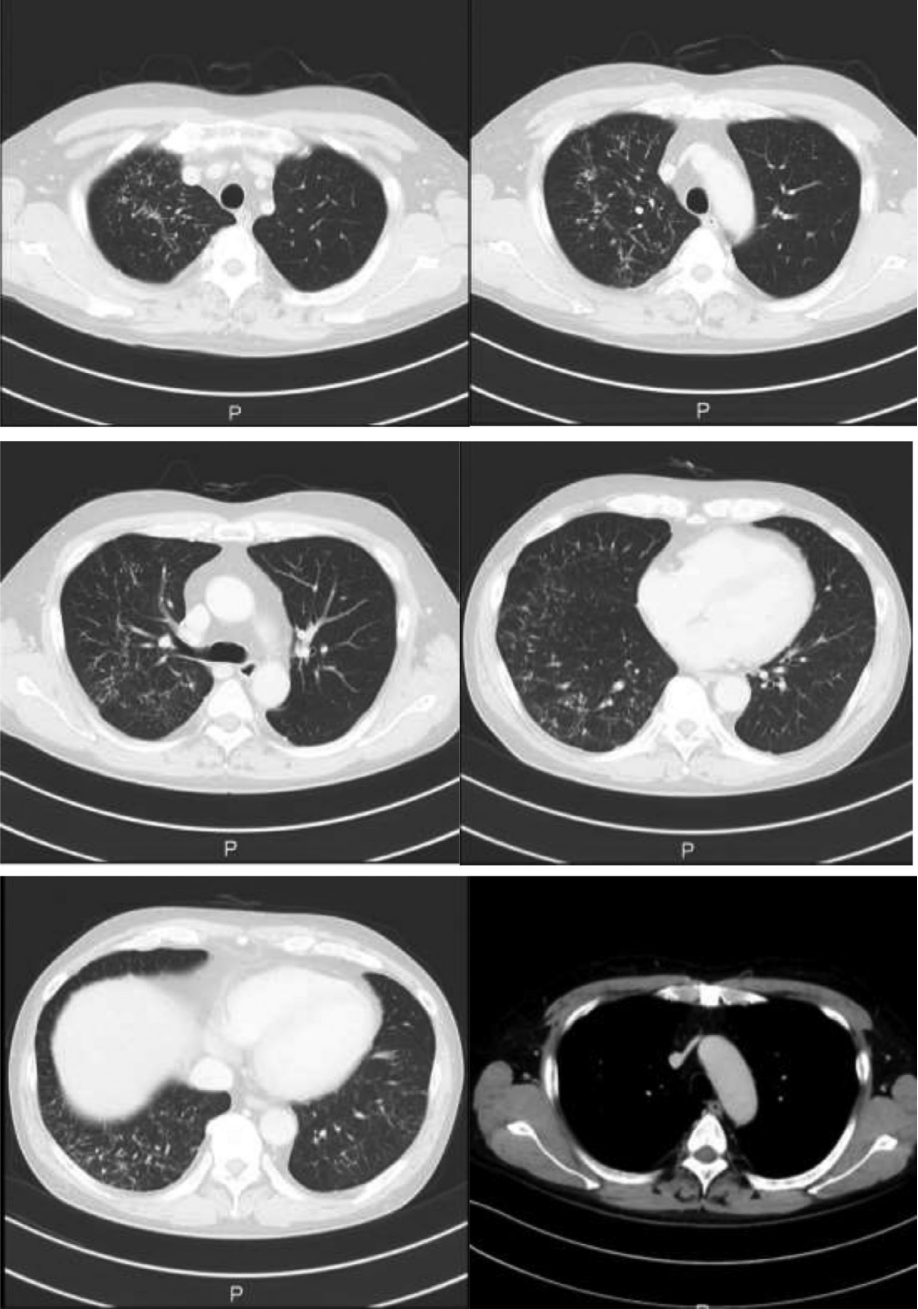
Computed tomography (CT) images demonstrated diffuse irregular nodules bilaterally.
